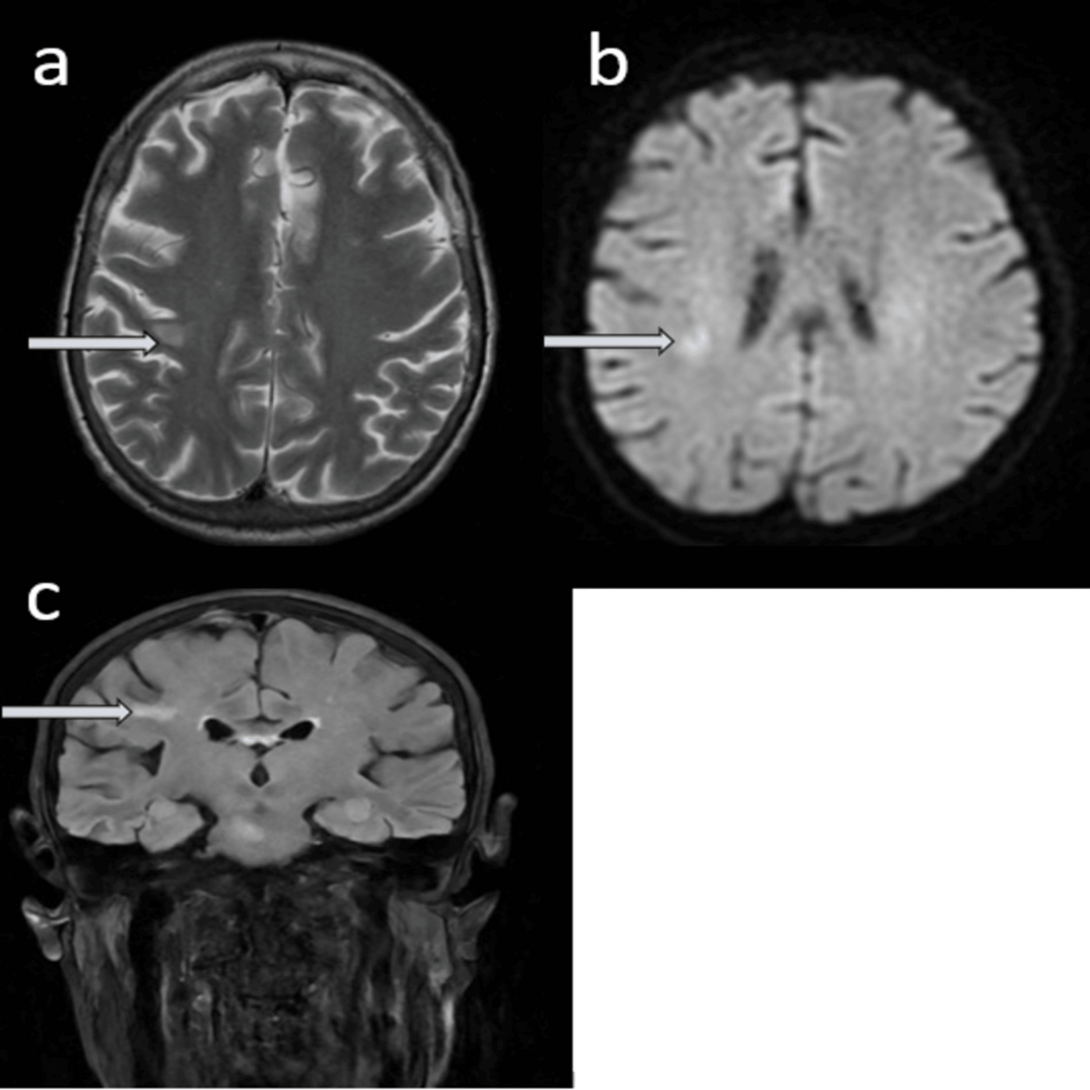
MRI head without contrast showing interval resolution of PRES. (a) T2W image, axial section; (b) DWI image, axial section; (c) FLAIR image, coronal view. DWI: diffusion-weighted imaging; FLAIR: fluid-attenuated inversion recovery.
